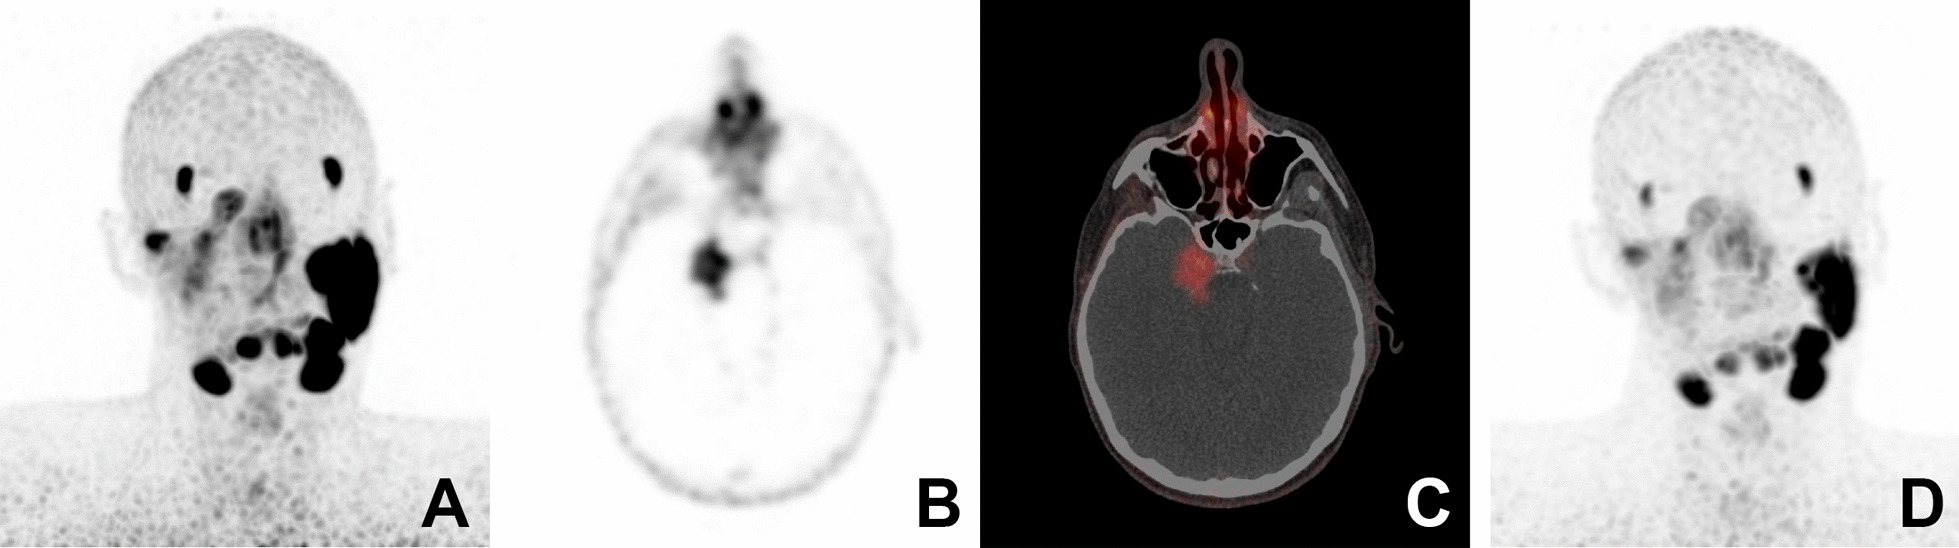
Patient no. 2 suffering from recurrent and metastatic AdCC of the right parotid gland. Imaging depicts moderate PSMA-ligand uptake in the recurrent parapharyngeal and intracranial tumour localizations: before (A, B and C) and after (D) therapy. A Coronal maximum intensity projection (before therapy SUVmax 6.5); B axial PET; C axial PET/CT reconstruction; D coronal maximum intensity projection (after therapy SUVmax 4.5)
